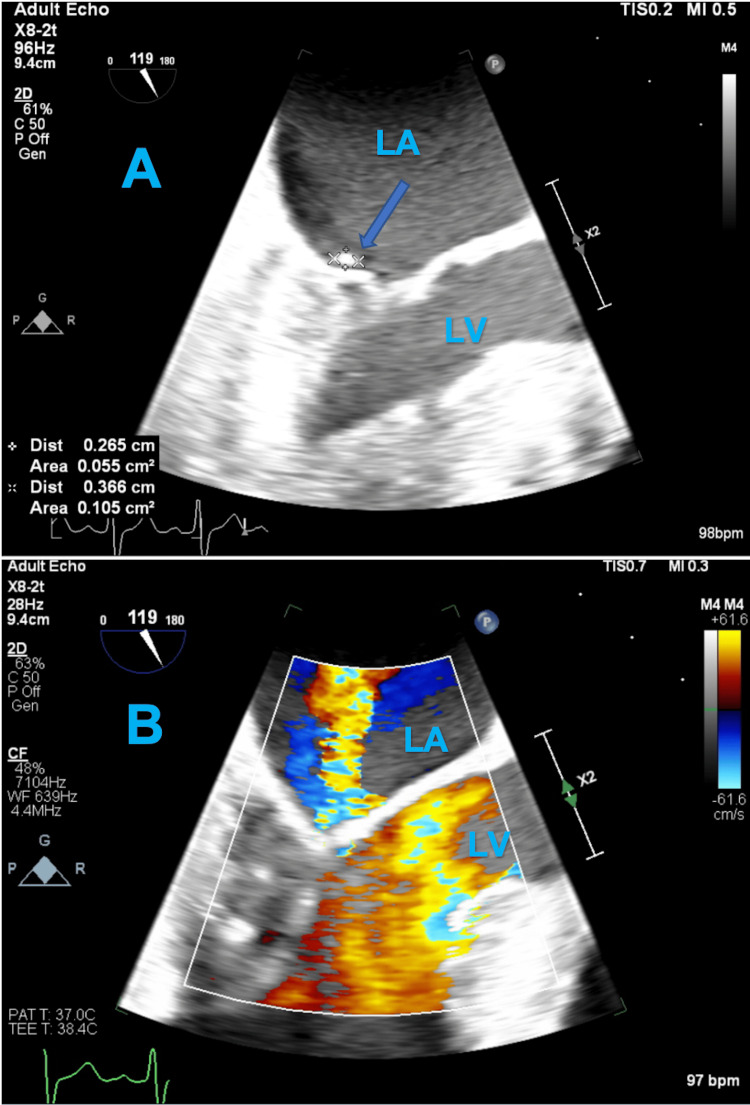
Transesophageal echocardiography (TEE) findings. (A) TEE showing a 0.27 cm x 0.37 cm sessile vegetation on the body of the posterior leaflet of the mitral valve (blue arrow). (B) TEE showing moderate to severe mitral regurgitation. TEE: Transesophageal echocardiography; LA: Left atrium; LV: Left ventricle.
